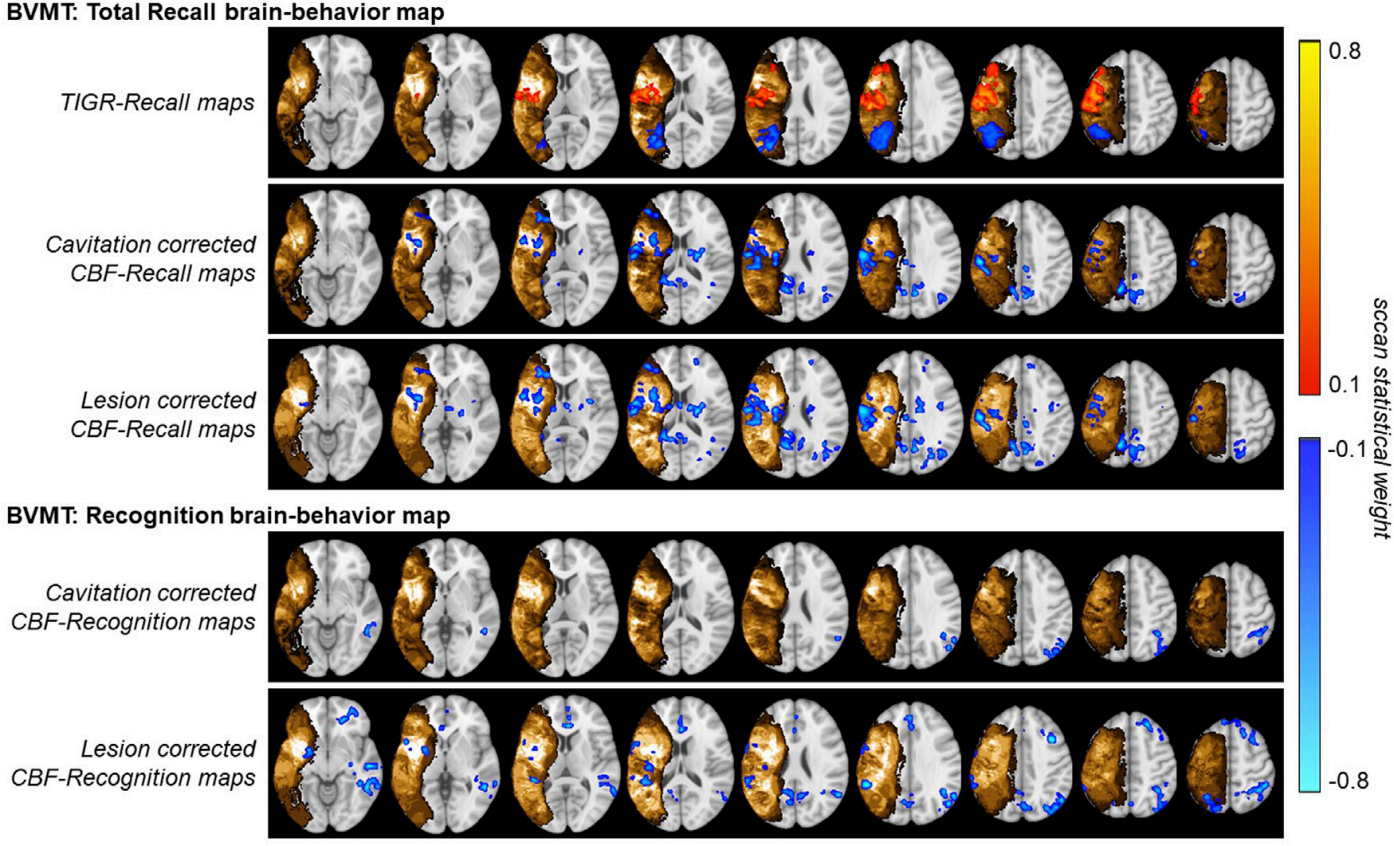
Multivariate brain-behavior maps using TIGR MRI and either cavitation-corrected or lesion-corrected cerebral blood flow (CBF) for BVMT total recall and BVMT recognition. The gold indicates either mean TIGR (cavitation corrected) or lesion overlap (lesion corrected). The red and blue indicate regions of significant multivariate brain-behavior relationships.
